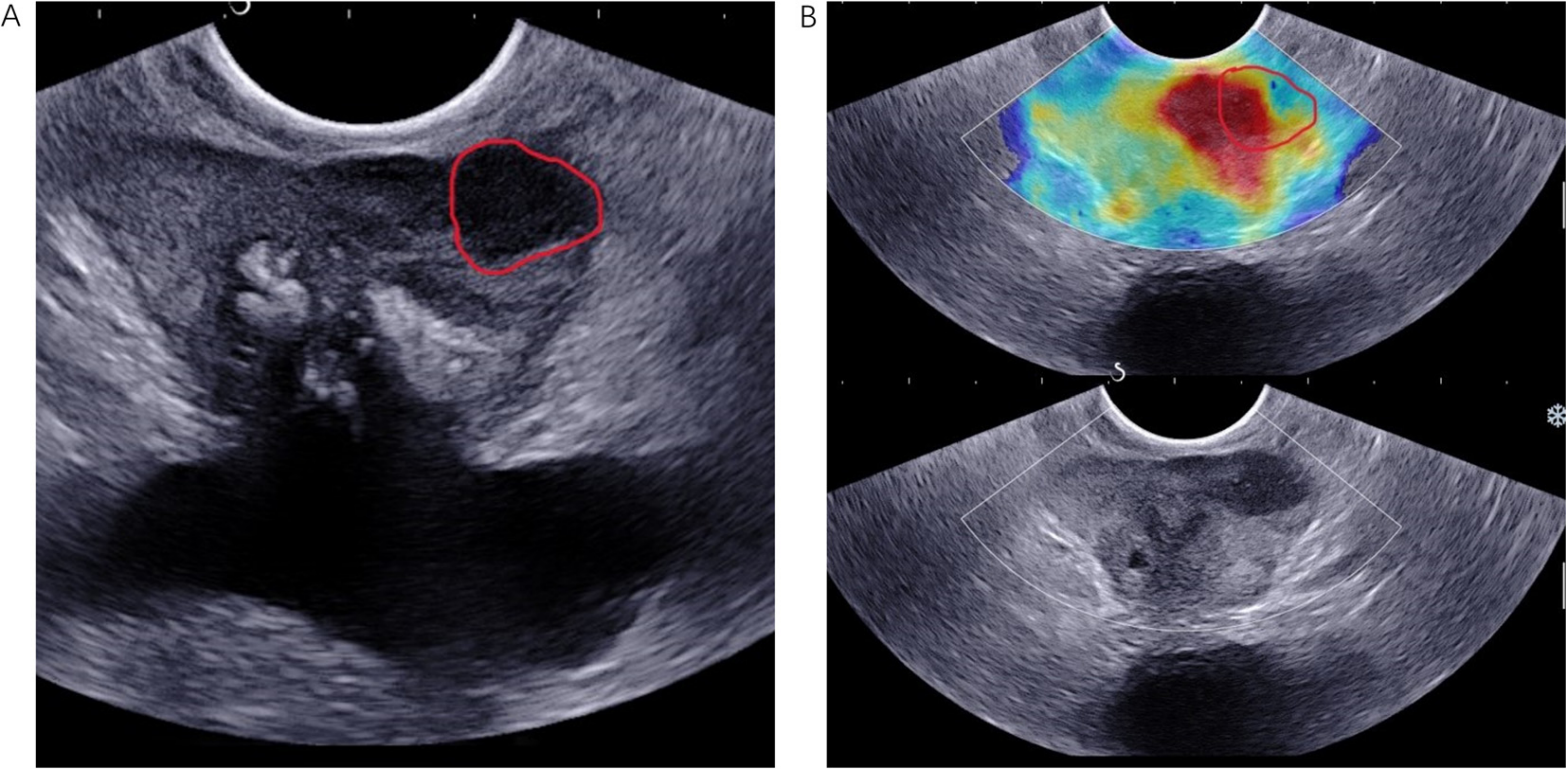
TRUS B-mode imaging (A) and SWE imaging (B) were conducted from the same anatomical location in an 83-year-old patient diagnosed with PCa, characterized by a fPSA level of 0.78 ng/mL, a tPSA level of 13.7 ng/mL, and a biopsy Gleason score of 4 + 3 = 7. The ROI, delineated by a red solid line, was identified in both the B-mode ultrasound and SWE images. PCa, prostate cancer.
